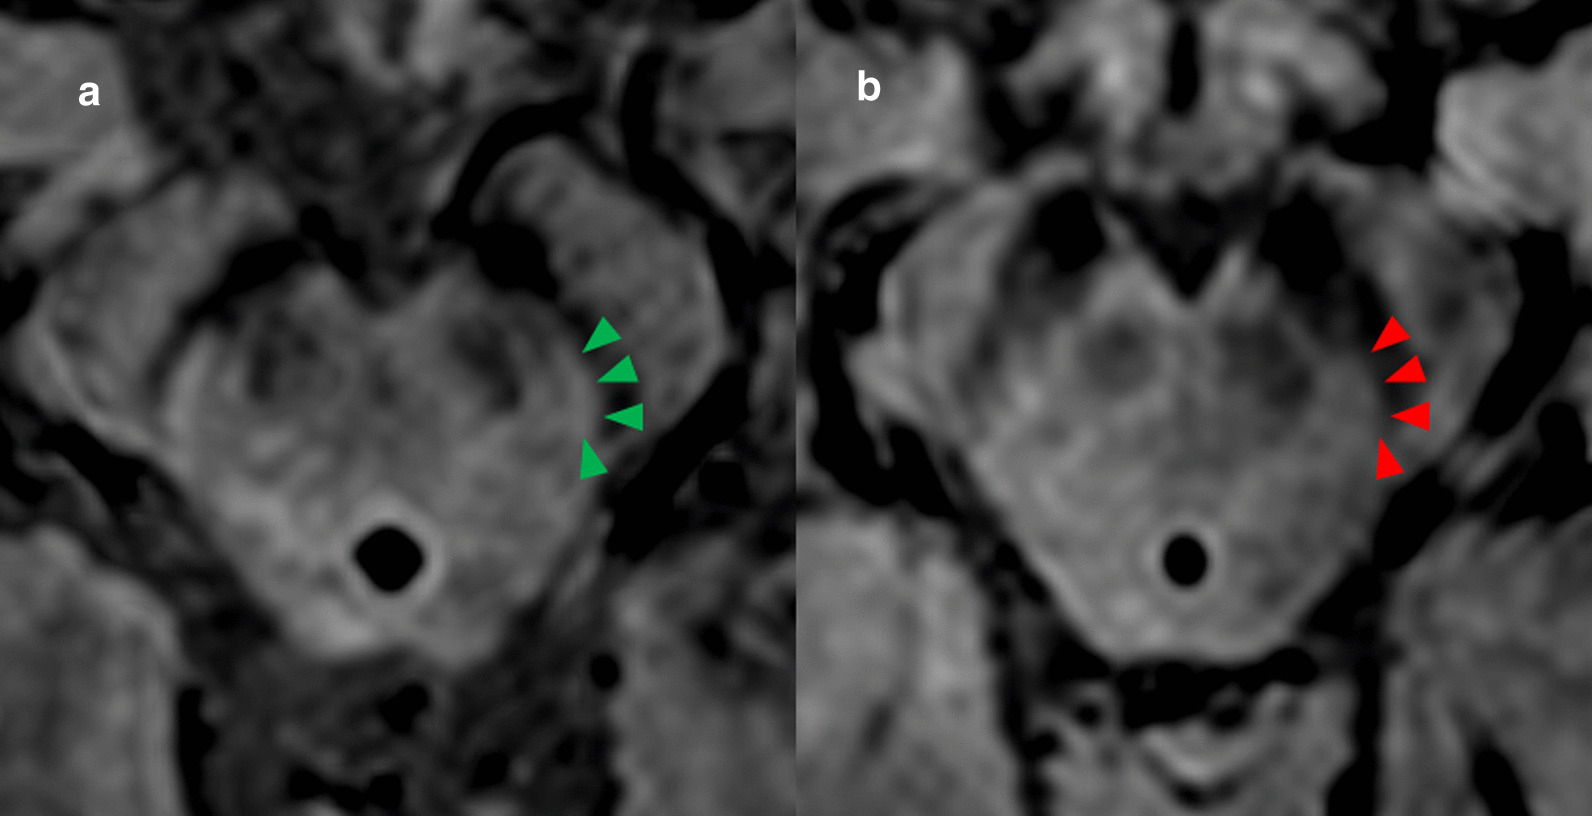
Evaluation of Nigrosome 1 in high-resolution MRI. Nigrosome 1 assessment of the substantia nigra in axial plane susceptibility weighted imaging MRI of the midbrain. Green arrows highlight the presence of Nigrosome 1 in a 62-year-old man (a). Red arrows highlight the absence of Nigrosome 1 in substantia nigra in a 65-year-old woman (b)
